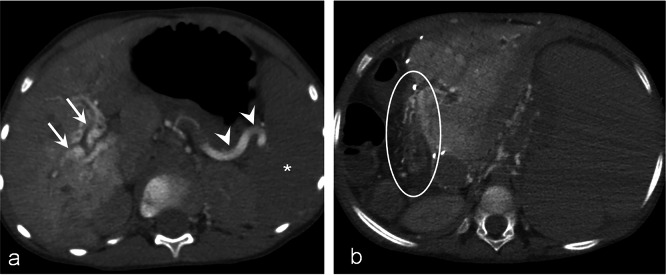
Computed tomography arterioportography (CT AP) findings in two different children. a A 5-year-old male patient with portal vein thrombosis. Axial images obtained after contrast injection in the superior mesenteric artery detect portal biliopathy (arrows) and reversed blood flow in the splenic vein (arrowheads). The spleen does not show contrast enhancement (white asterisk). b An 8-year-old male patient with portal vein thrombosis after liver transplantation. CT AP shows varices and diffuse enhancement of the hepaticojejunostomy (ellipse)
